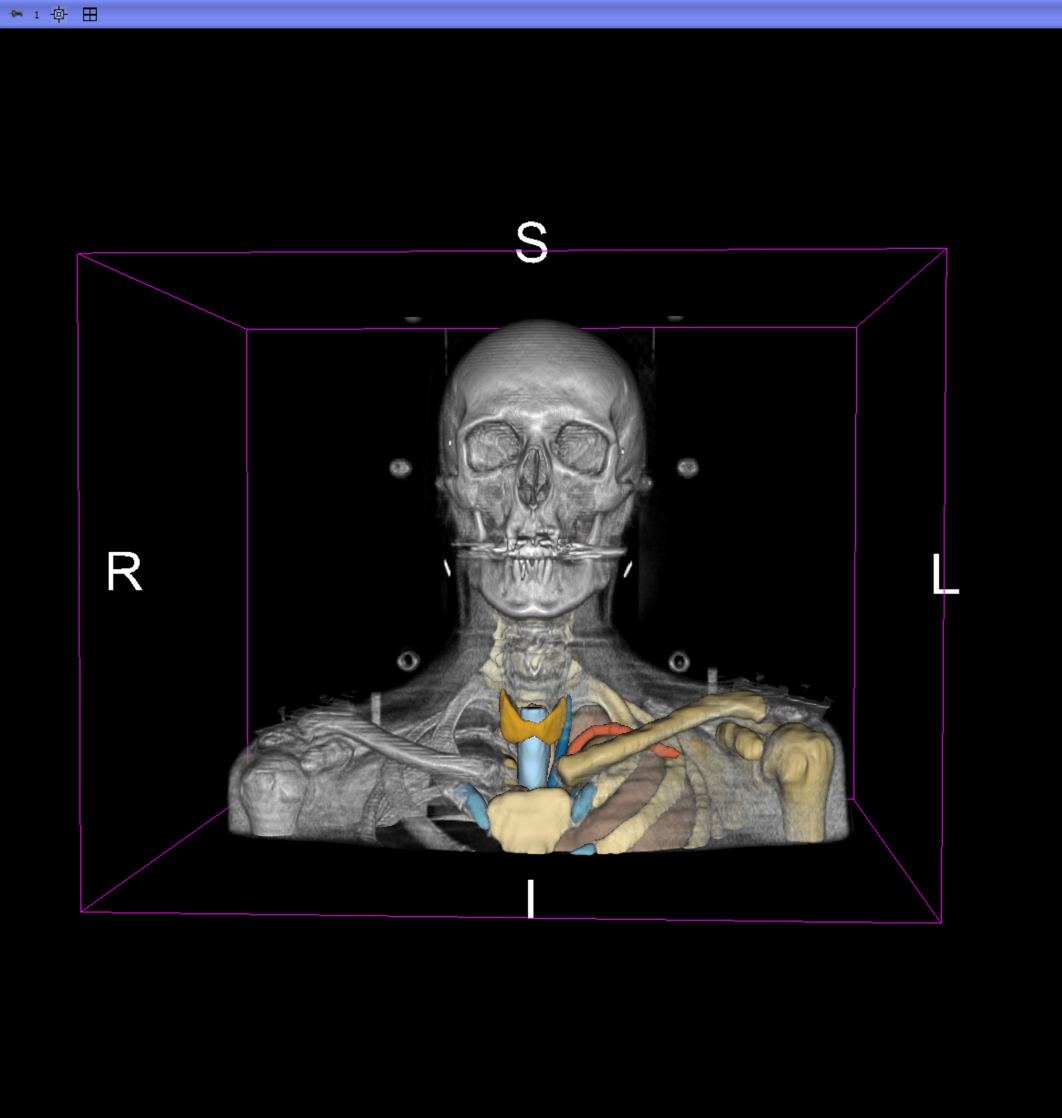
Segmentation with TotalSegmentator in 3D Slicer Volumetric rendering in 3D Slicer of an anonymized head‑and‑neck CT segmented with TotalSegmentator ("total," "headneck_bones," "headneck_muscles"); for visual clarity, only selected left‑sided segments and central midline structures are displayed, including the thyroid, to provide inputs for subsequent 3D model generation.
